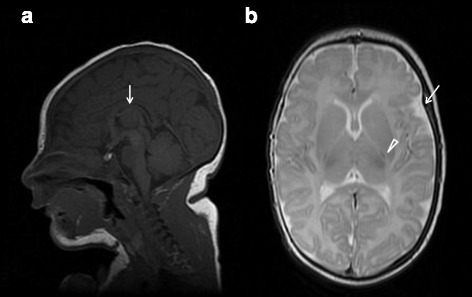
Brain MRI at age 18 days. Sagittal T1WI view showing thinning of the corpus callosum (arrow), with anterior predominance, and some degree of frontal lobe volume reduction (a). Axial T2WI view showing asymmetric enlargement of temporal subarachnoidal spaces (arrow) and mildly prominent sulci over the cerebral convexity, suggesting incipient atrophy. Myelination is delayed with minimal signals observed at the posterior limb of the internal capsule (arrowhead) and corpus callosum (b)
